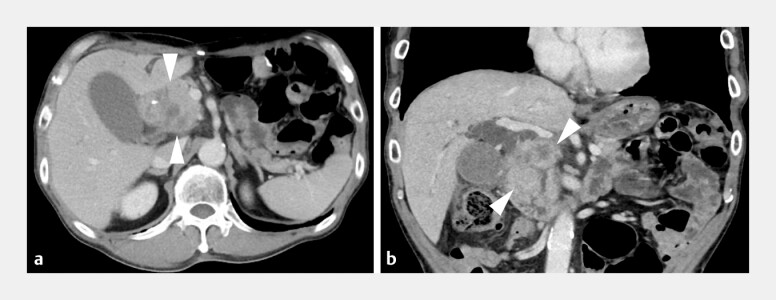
Computed tomography images show huge lymph node metastases (arrowheads) that obstruct
